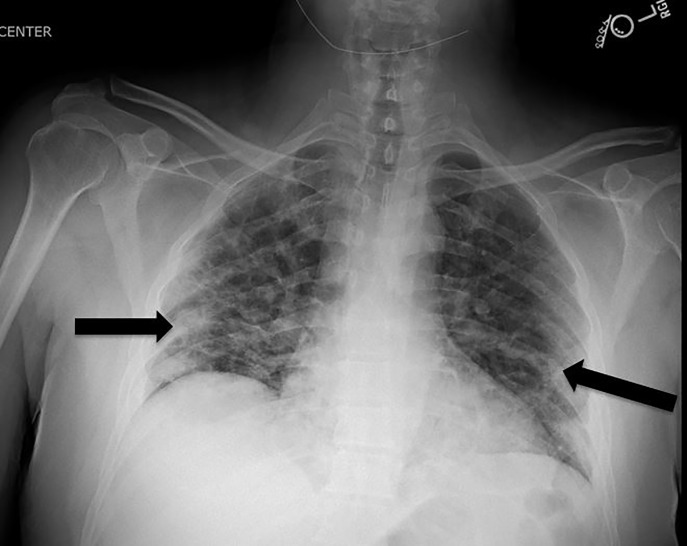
Chest radiograph of the patient. Note bilateral patchy opacities, most prominently at periphery of the lung concerning for multifocal pneumonia (arrows).
